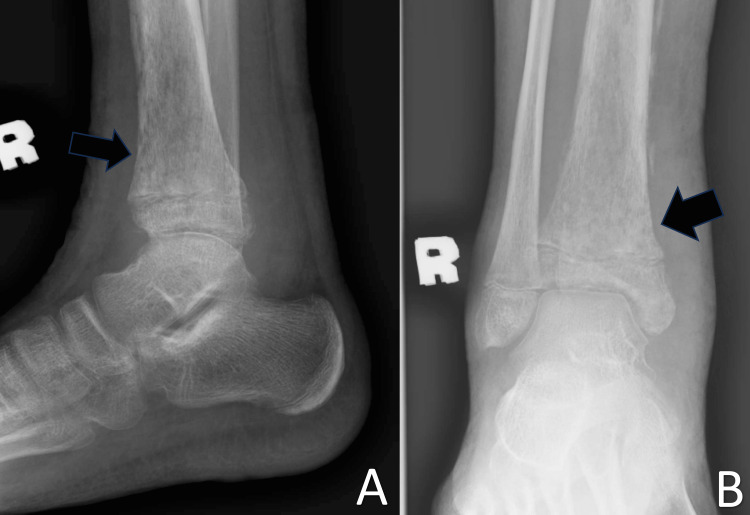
Plain radiograph of the right ankle in anteroposterior (A) and lateral (B) views The images revealed loss of bony trabeculae and osteolysis changes (black arrows)
